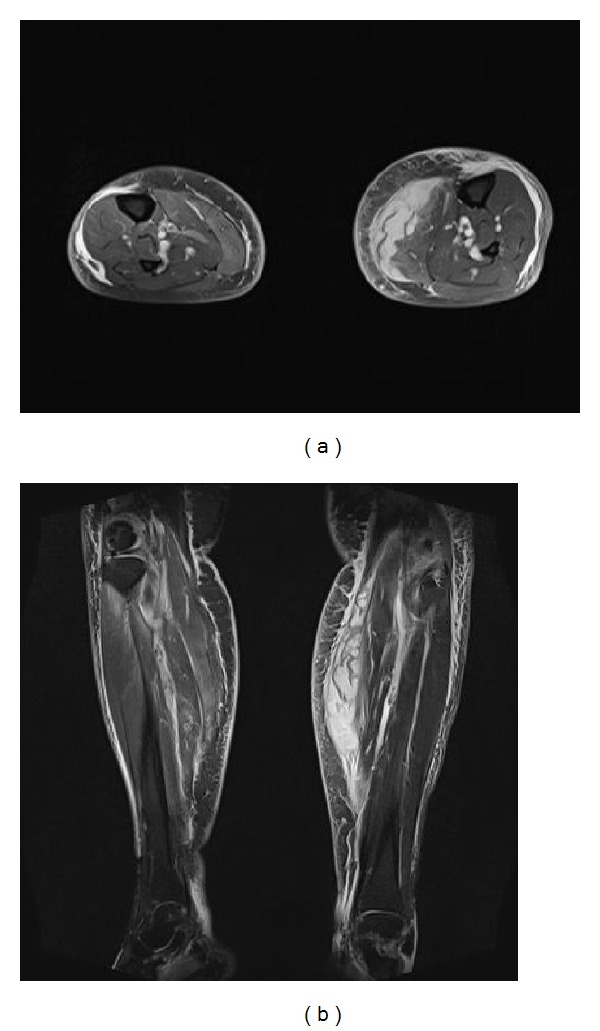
Postoperative T1-weighted MR images of both crures, revealing fluid collection in the posterior compartment.
